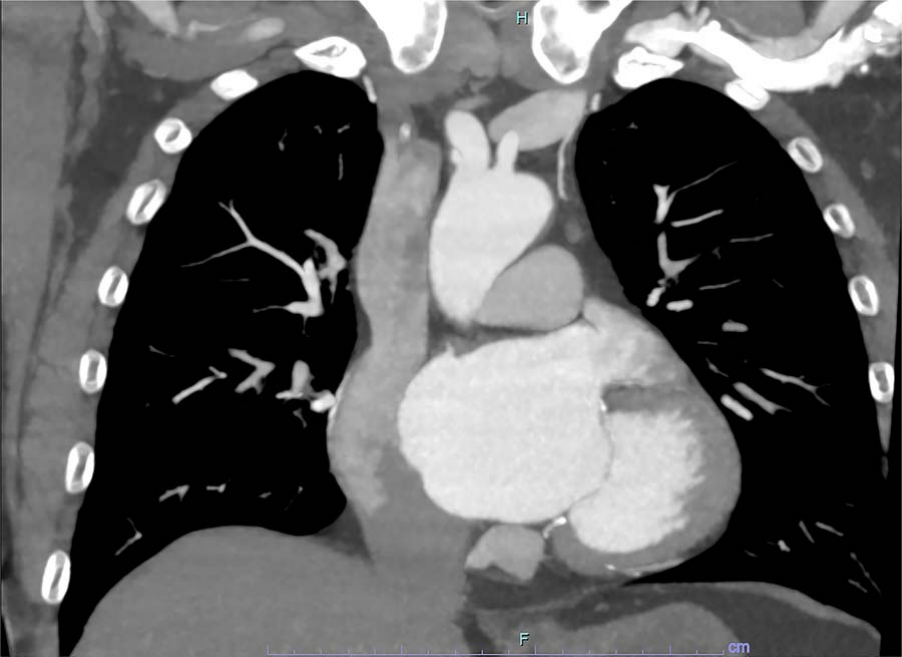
Coronal slice from CT aortogram demonstrating the origin of the dissection flap.
